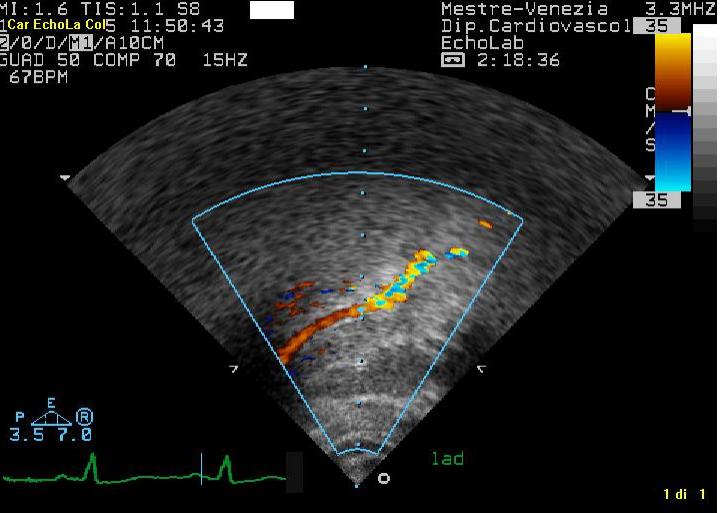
represents the Ultrasound findings of distal left anterior highlighted with color-Doppler.
